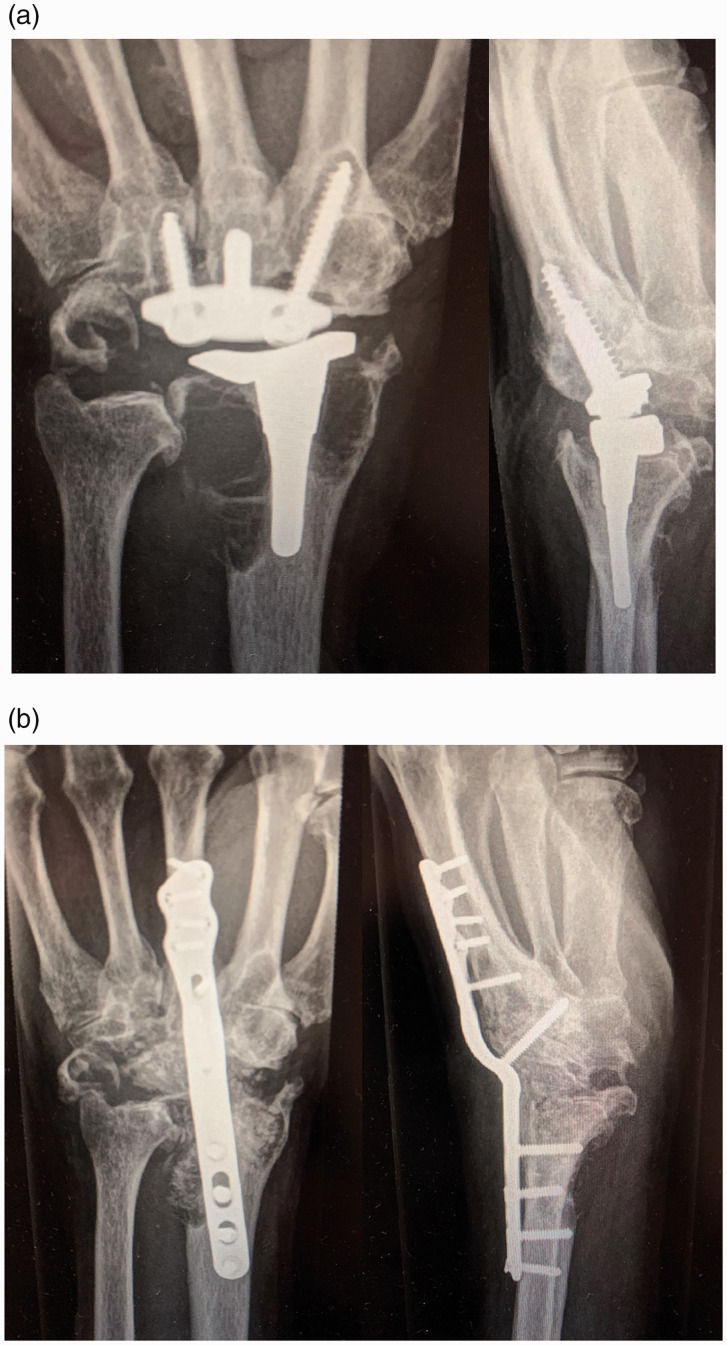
Lateral and anteroposterior radiographs of (a) a failed Universal 2 wrist implant and (b) after revision to total wrist arthrodesis.
